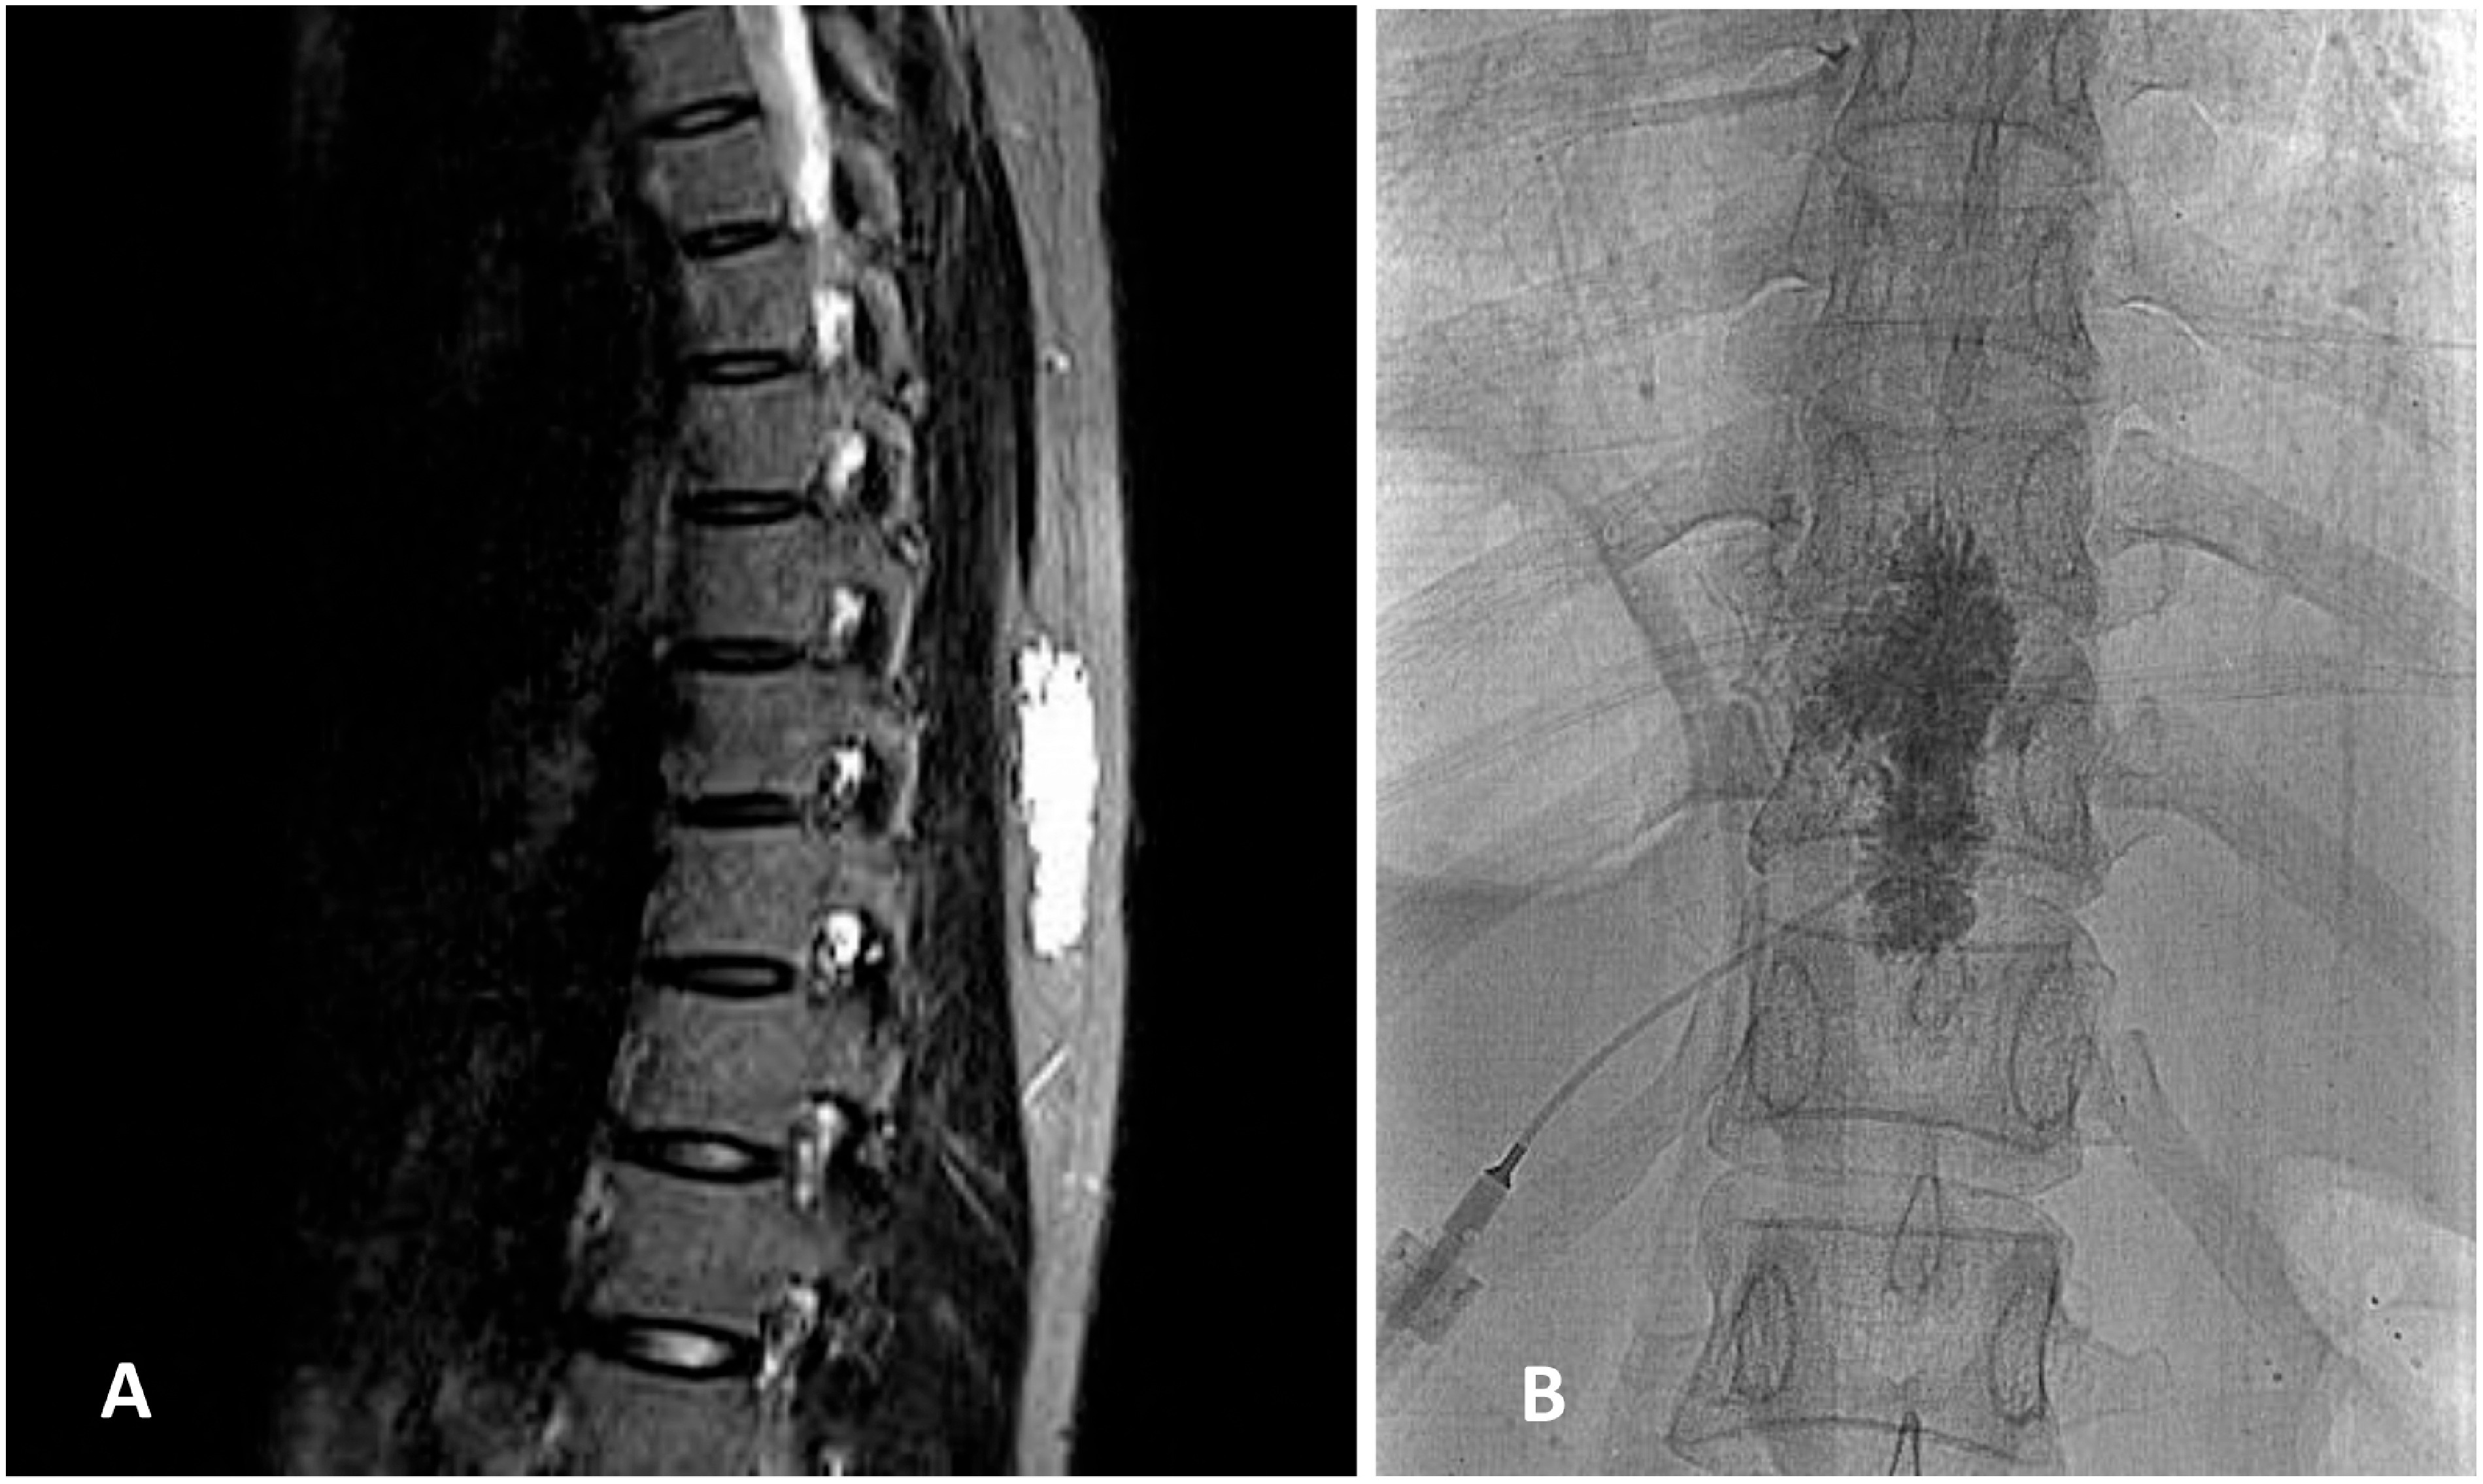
Fig. 1 A 26-year-old female patient experienced swelling and a painful mass in her back for many years. (A) MRI of the venous malformation. (B) DSA image shows a small drainage vein under the skin that did not drain into the medullary vein. This malformation was classed as type II according to Puig et al.^8)^
